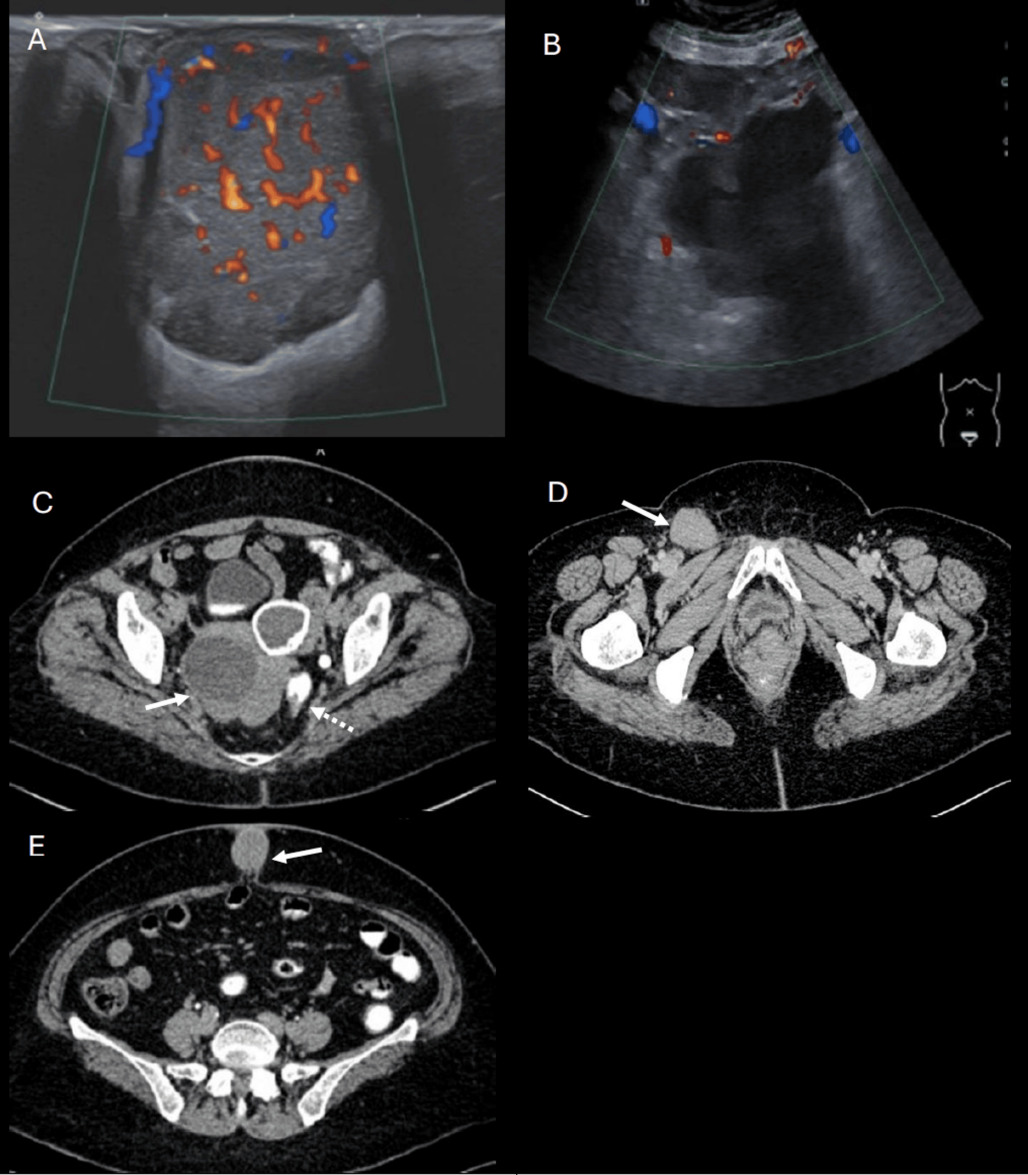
Ultrasound of the umbilicus (A) and abdomen (B) revealed a well-defined, vascularized, heterogeneous hypoechoic lesion within the umbilicus and a suspicious adnexal mass, respectively. Contrast-enhanced CT of the abdomen (axial view) confirmed the presence of a right complex adnexal mass (arrow, C), displacing the adjacent rectal and sigmoid colon (dash arrow, C) with poor fat demarcation. The enlarged right inguinal lymph node, measuring 2.4 x 3.6 cm, showed loss of normal fatty hilum (arrow, D). An enhancing soft tissue umbilical lesion (arrow, E) indicative of metastatic carcinoma was also evident.
